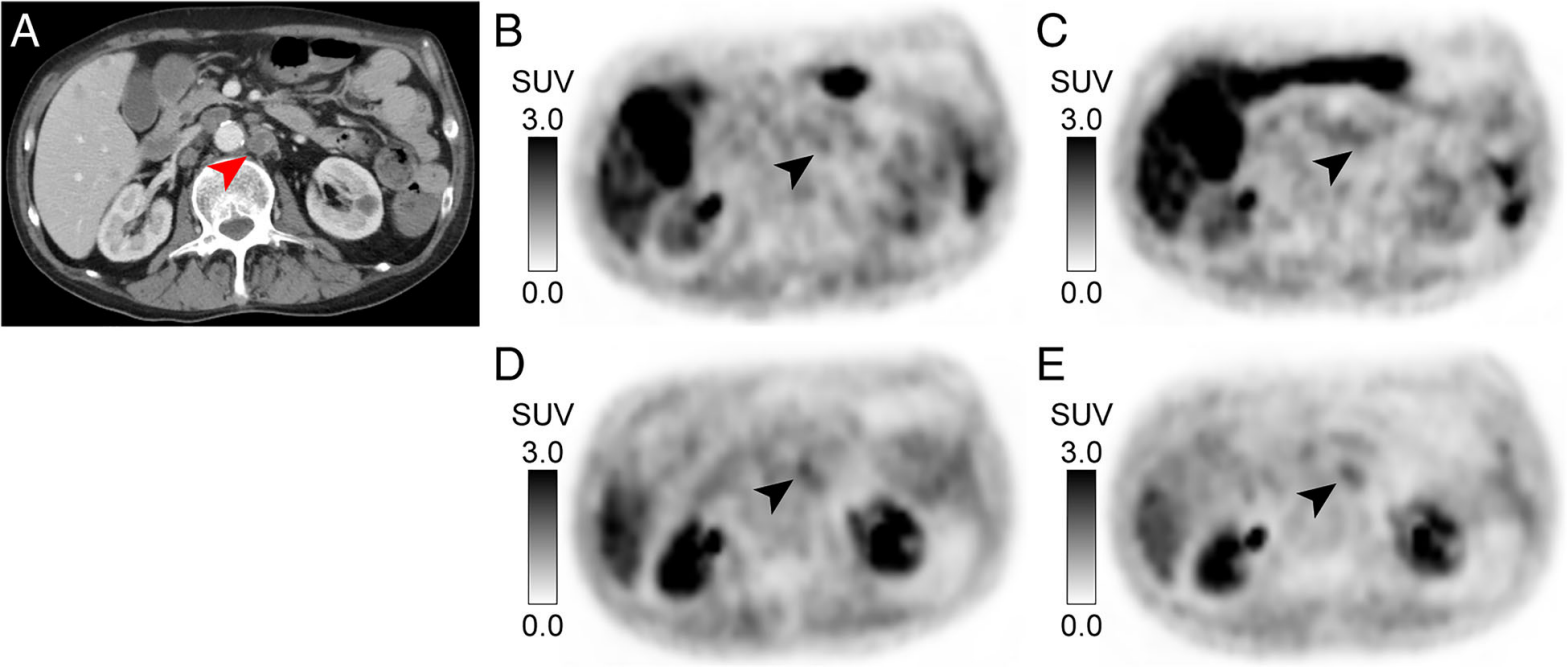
Representative images of a patient. a Transaxial image of contrast-enhanced CT. The swollen paraaortic lymph node is shown. b, c 2-h and 4-h images with [^18^F]FMISO. d, e 1-h and 2-h images with [^18^F]DiFA. Tracer uptake to the lymph node metastasis of rectal adenocarcinoma is clearly detected in all images. The contrast at 1 h and 2 h with [^18^F]DiFA (TMR = 1.25 and 1.37, respectively) is better than that at 2 h with [^18^F]FMISO (TMR = 1.09) and equivalent to 4-h [^18^F]FMISO (TMR = 1.44)
